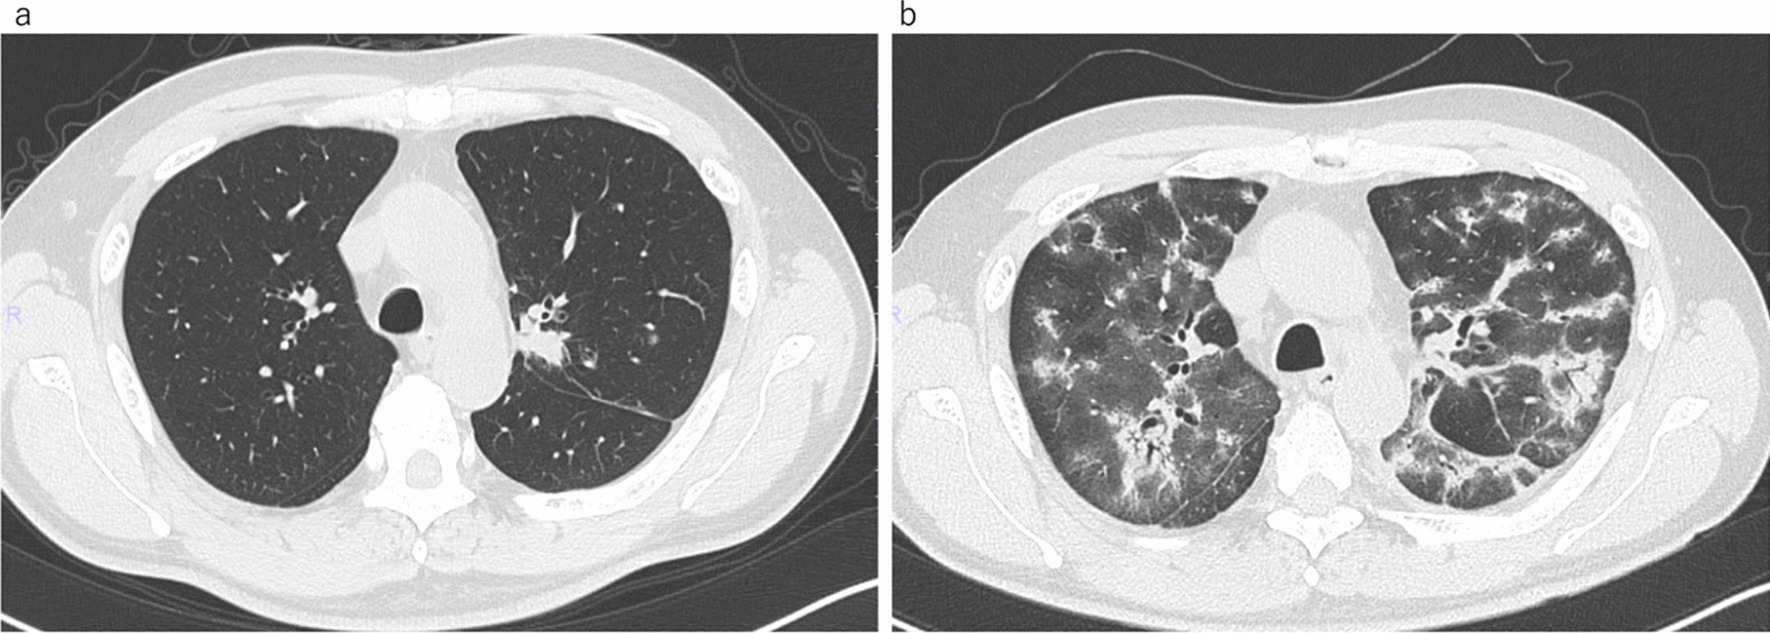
Thin-slice CT before and after the onset of DILD. Prior to the onset of DILD (a), a shadow of the primary lesion was observed in the left upper lobe of the lung. No abnormal shadow was seen in the lung field. After the patient developed DILD (b), the primary lesion was observed to be shrinking. However, ground-glass opacities and infiltrations were seen spreading on both sides. *CT* computed tomography, *DILD* drug-induced interstitial lung disease
